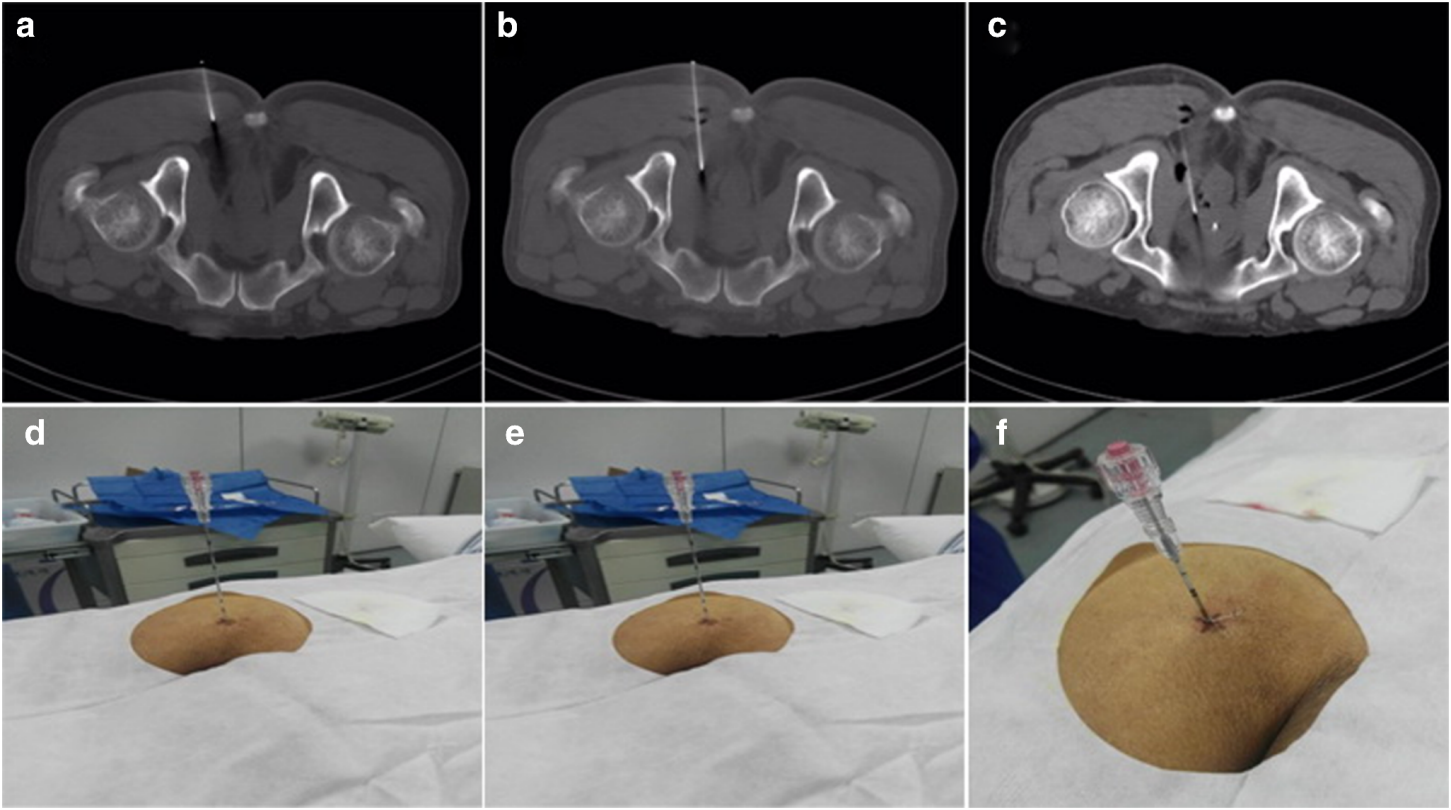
^68^Ga-PSMA-TB was performed in the prone position, using a single puncture percutaneous transgluteal approach technique. The needle was introduced into the gluteus maximus (a and d), the ischial anal fossa (b and e) and intra-prostatic lesion (c and f) under CT guidance in real-time
